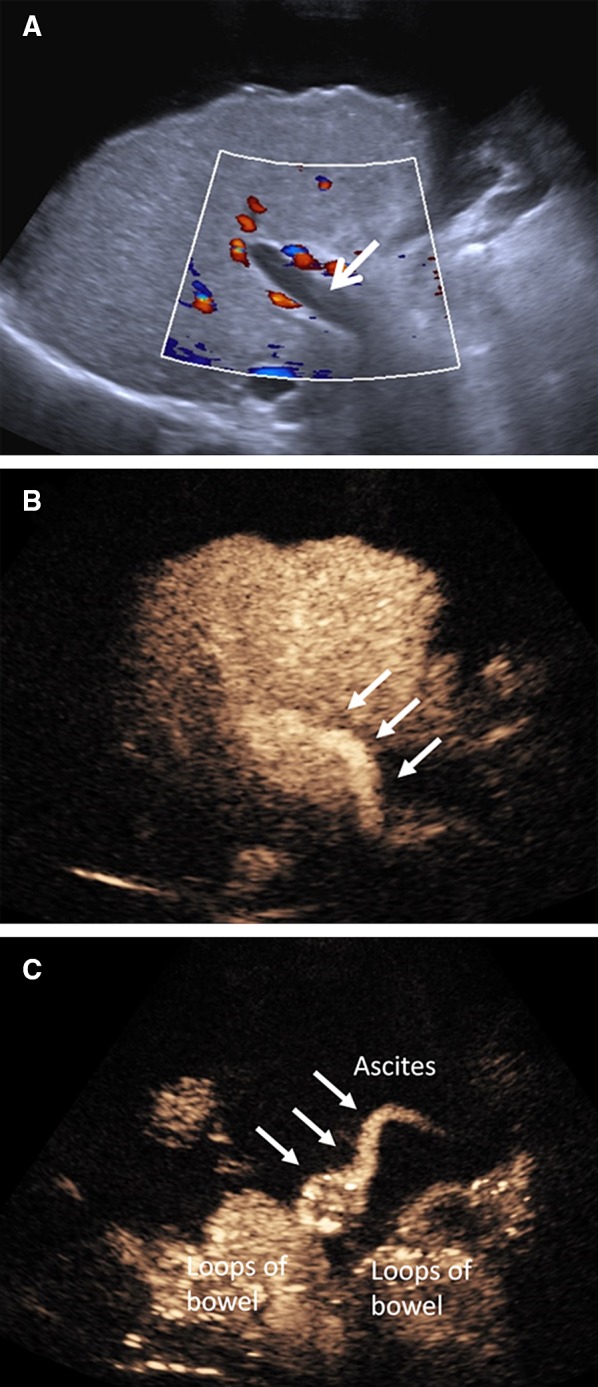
A 66-year-old male with liver cirrhosis developed hepatorenal syndrome. Color Doppler US failed to demonstrate the presence of portal flow likely due to slow flow (A, arrow). CEUS was performed instead of CECT due to poor renal function. CEUS demonstrated a patent portal vein (B, arrows). Isolated CEUS image from the same patient shows recanalisation of large umbilical vein (C, arrows) surrounded by large volume ascites (black area) in keeping with established portal hypertension
